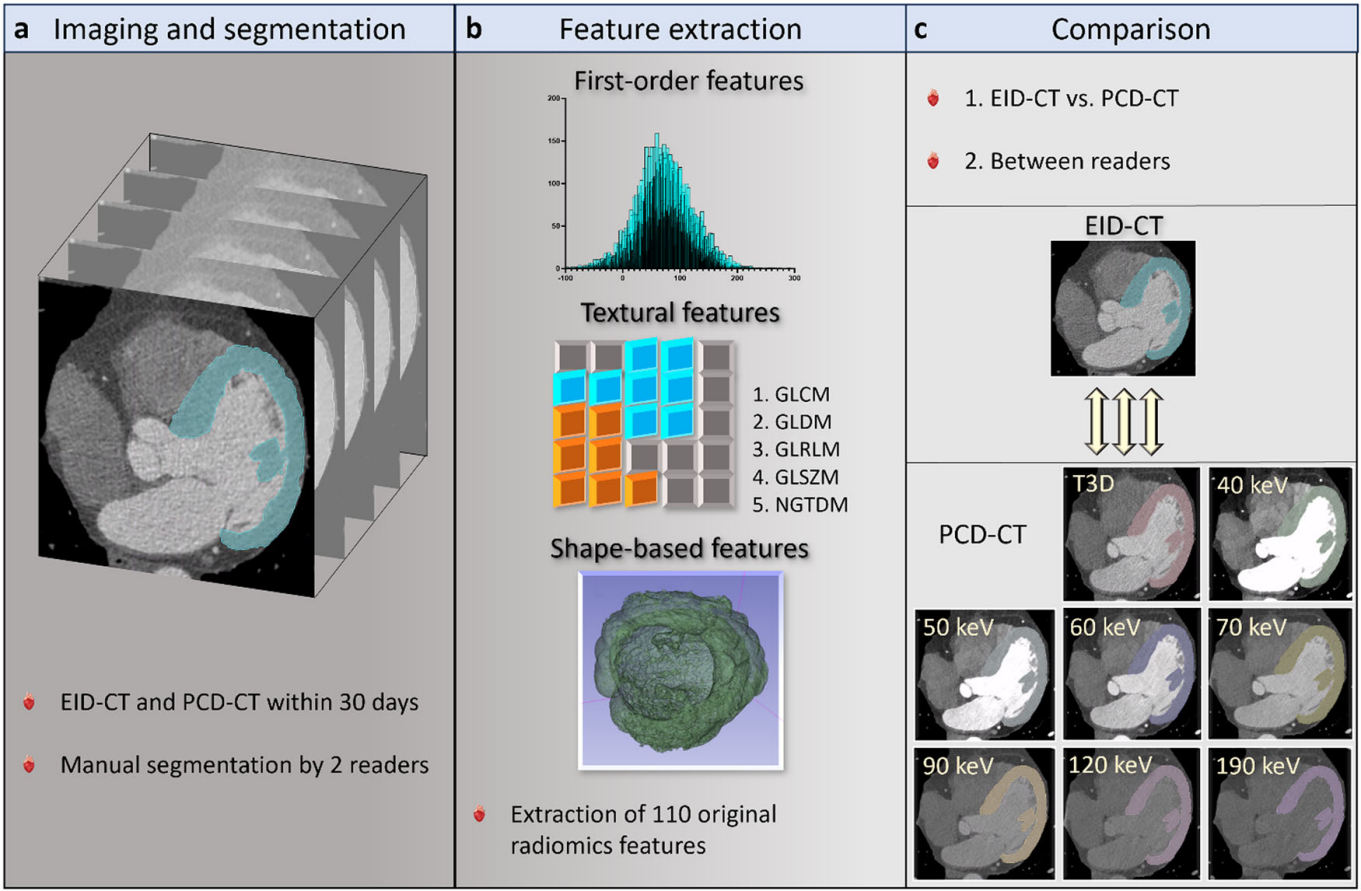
The overall acquisition, processing, and analysis framework of the datasets. Both EID-CT and PCD-CT datasets of enrolled patients were segmented (a) by two readers. A total of 18 first-order, 75 textural, and 17 shape-based radiomic features were extracted (b) from the segmented volume of interests. These values were then compared (c) between EID-CT and PCD-CT reconstructions, among different VMIs, and between different segmentations to assess inter-reader agreement. EID-CT, Energy-integrating detector CT; GLCM, Gray level co-occurrence matrix; GLDM, Gray level dependency matrix; GLRLM, Gray level run length matrix; GLSZM, Gray level size zone matrix; NGTDM, Neighboring gray-tone difference matrix; PCD-CT, Photon-counting detector CT; VMI, Virtual monoenergetic image
